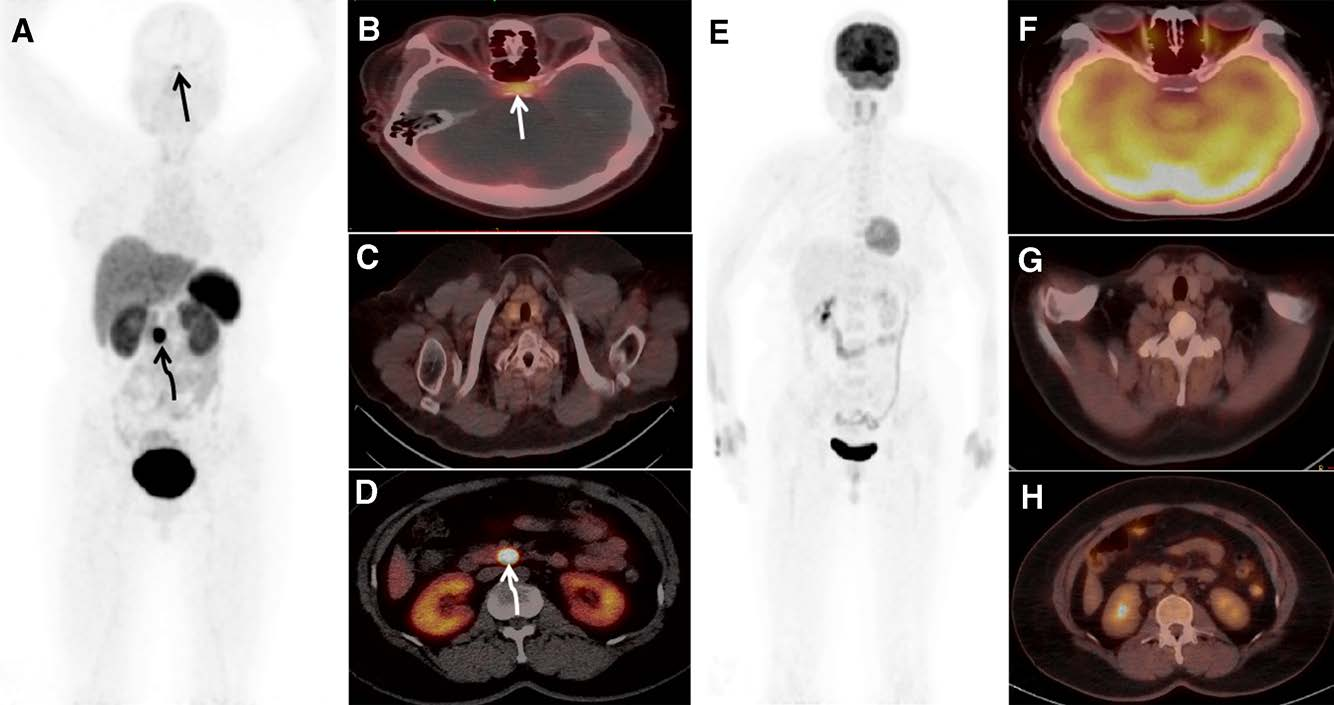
^68^Ga-DOTATATE PET/CT images (A–D) and ^18^F-FDG PET/CT images (E–H). (A) The MIP and axial fusion images of the ^68^Ga-DOTATATE PET/CT showing 1 focal areas of increased radiotracer uptake in the uncinate process of the pancreas (A and D, curved arrows) and a mild area of radiotracer uptake in the pituitary (A and B, thin arrows), whereas the parathyroid region was no significant abnormalities on PET/CT imaging (A and C). The MIP of ^18^F-FDG PET/CT and axial ^18^F-FDG PET/CT fusion images (E-H) showed no obvious abnormal ^18^F-FDG uptake. PET/CT = positron emission tomography/computed tomography.
